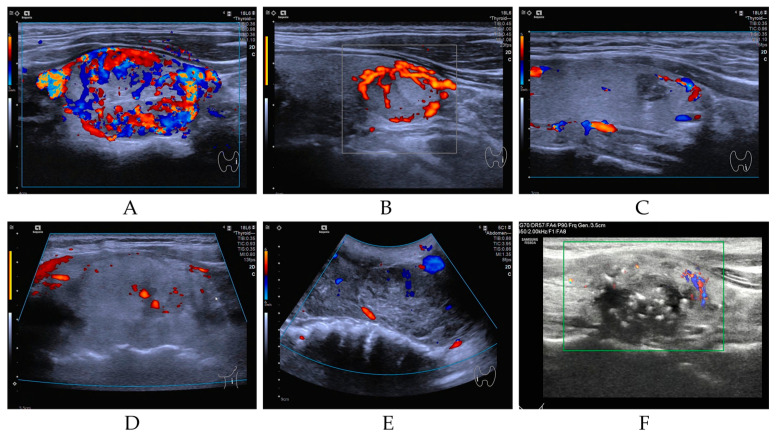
Representative ultrasonographic features of BT (A), LRN (B), PTC (C), FTC (D), PDTC&ATC (E), and MTC (F).
